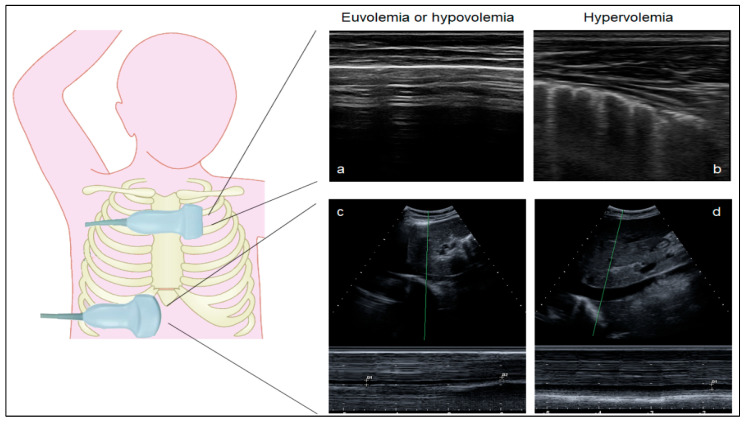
Ultrasound assessment of B-lines in different conditions of volume status. Ultrasound assessment of B-lines at lung ultrasound and inferior vena cava diameters in euvolemia or hypovolemia (a,c) and hypervolemia (b,d). (a) Normal lung: horizontal hyper-echogenic lines represent the lung pleura interface (A-lines). (b) Pulmonary congestion: hyperechogenic B-lines with a narrow base radiating from the transducer to the lower border of the window. Ultrasound of the inferior vena cava, measured on M-mode, is a sensitive method in volume status monitoring. The IVC is explored in the subxiphoid window in its sagittal view, measuring the maximum IVC diameter (IVCmax) during expiration and the minimum diameter during inspiration (IVCmin). The IVC collapsibility index (IVC-CI) is calculated using the standard formula [(IVCmax − IVCmin)/IVCmax] × 100. The IVC-CI inversely correlates with the central venous pressure. The negative thoracic pressure in inspiration increases the caval blood flow and decreases the intraluminal pressure. The venous return to the right atrium increases, accompanied by IVC emptying, and the diameter of the IVC decreases. (c) Hypovolemia: complete collapsibility of the IVC during inspiration. (d) Overhydration: insufficient collapsibility of the IVC during inspiration.
